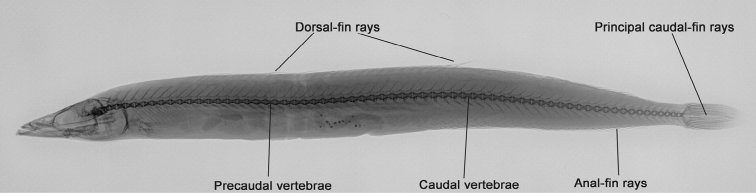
Radiograph of Common sand eel *Ammodytes
